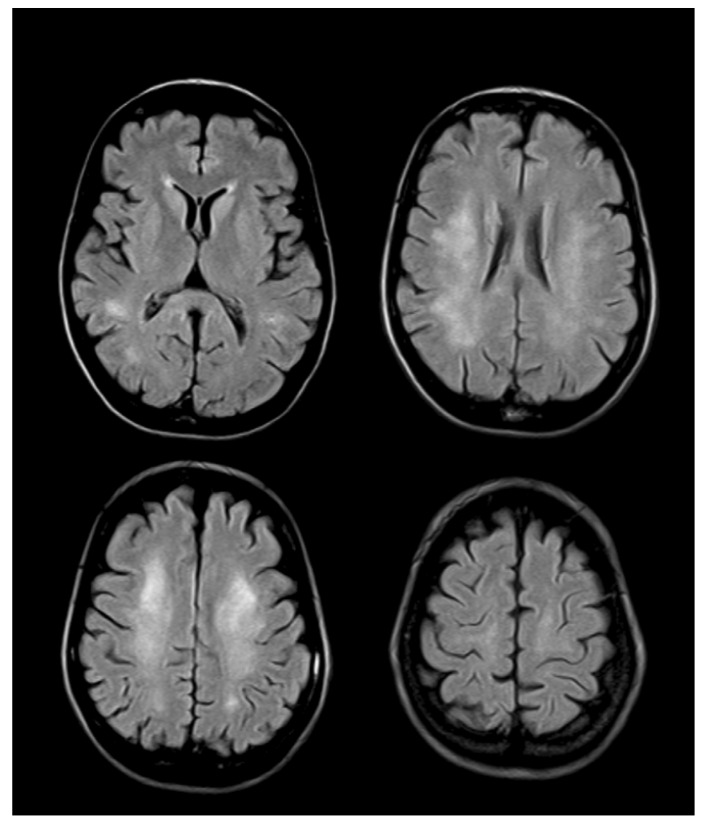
Brain MRI of a 45-year-old female presenting with slowly progressive cognitive impairment. Axial attenuated inversion recovery (FLAIR) images disclose diffuse and symmetric white matter signal hyperintensity.
